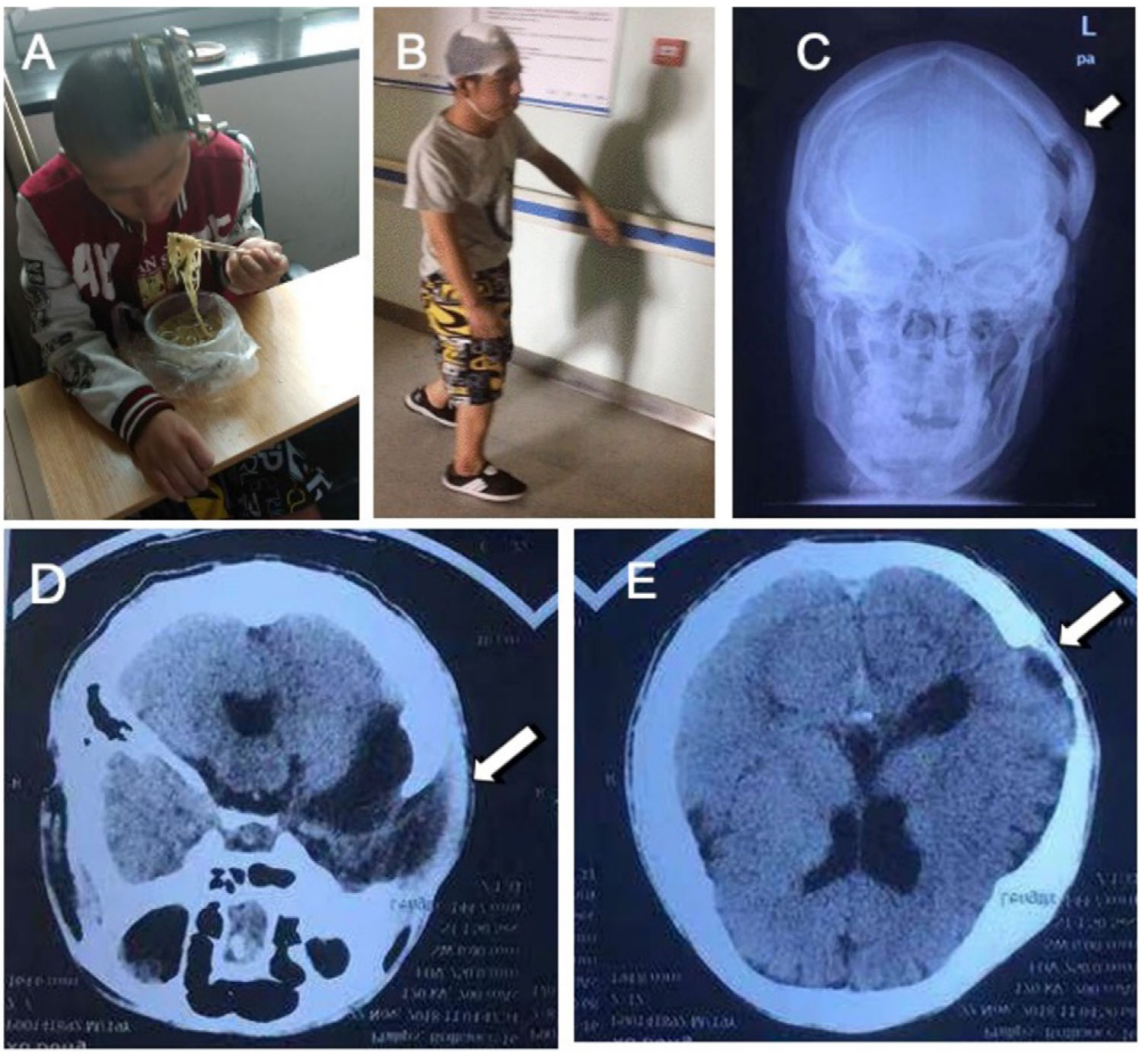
Therapeutic effects of CBT on case 1. A) Patient was able to feed himself 2 months after operation. B) He can walk slowly after removal of the external device. C) X‐ray results suggested bone transport repaired the bone gap. D) Representative CT Image of coronal position showed defects in temporal region were reduced. E) Representative CT Image of horizontal position showed bone defects were repaired.
